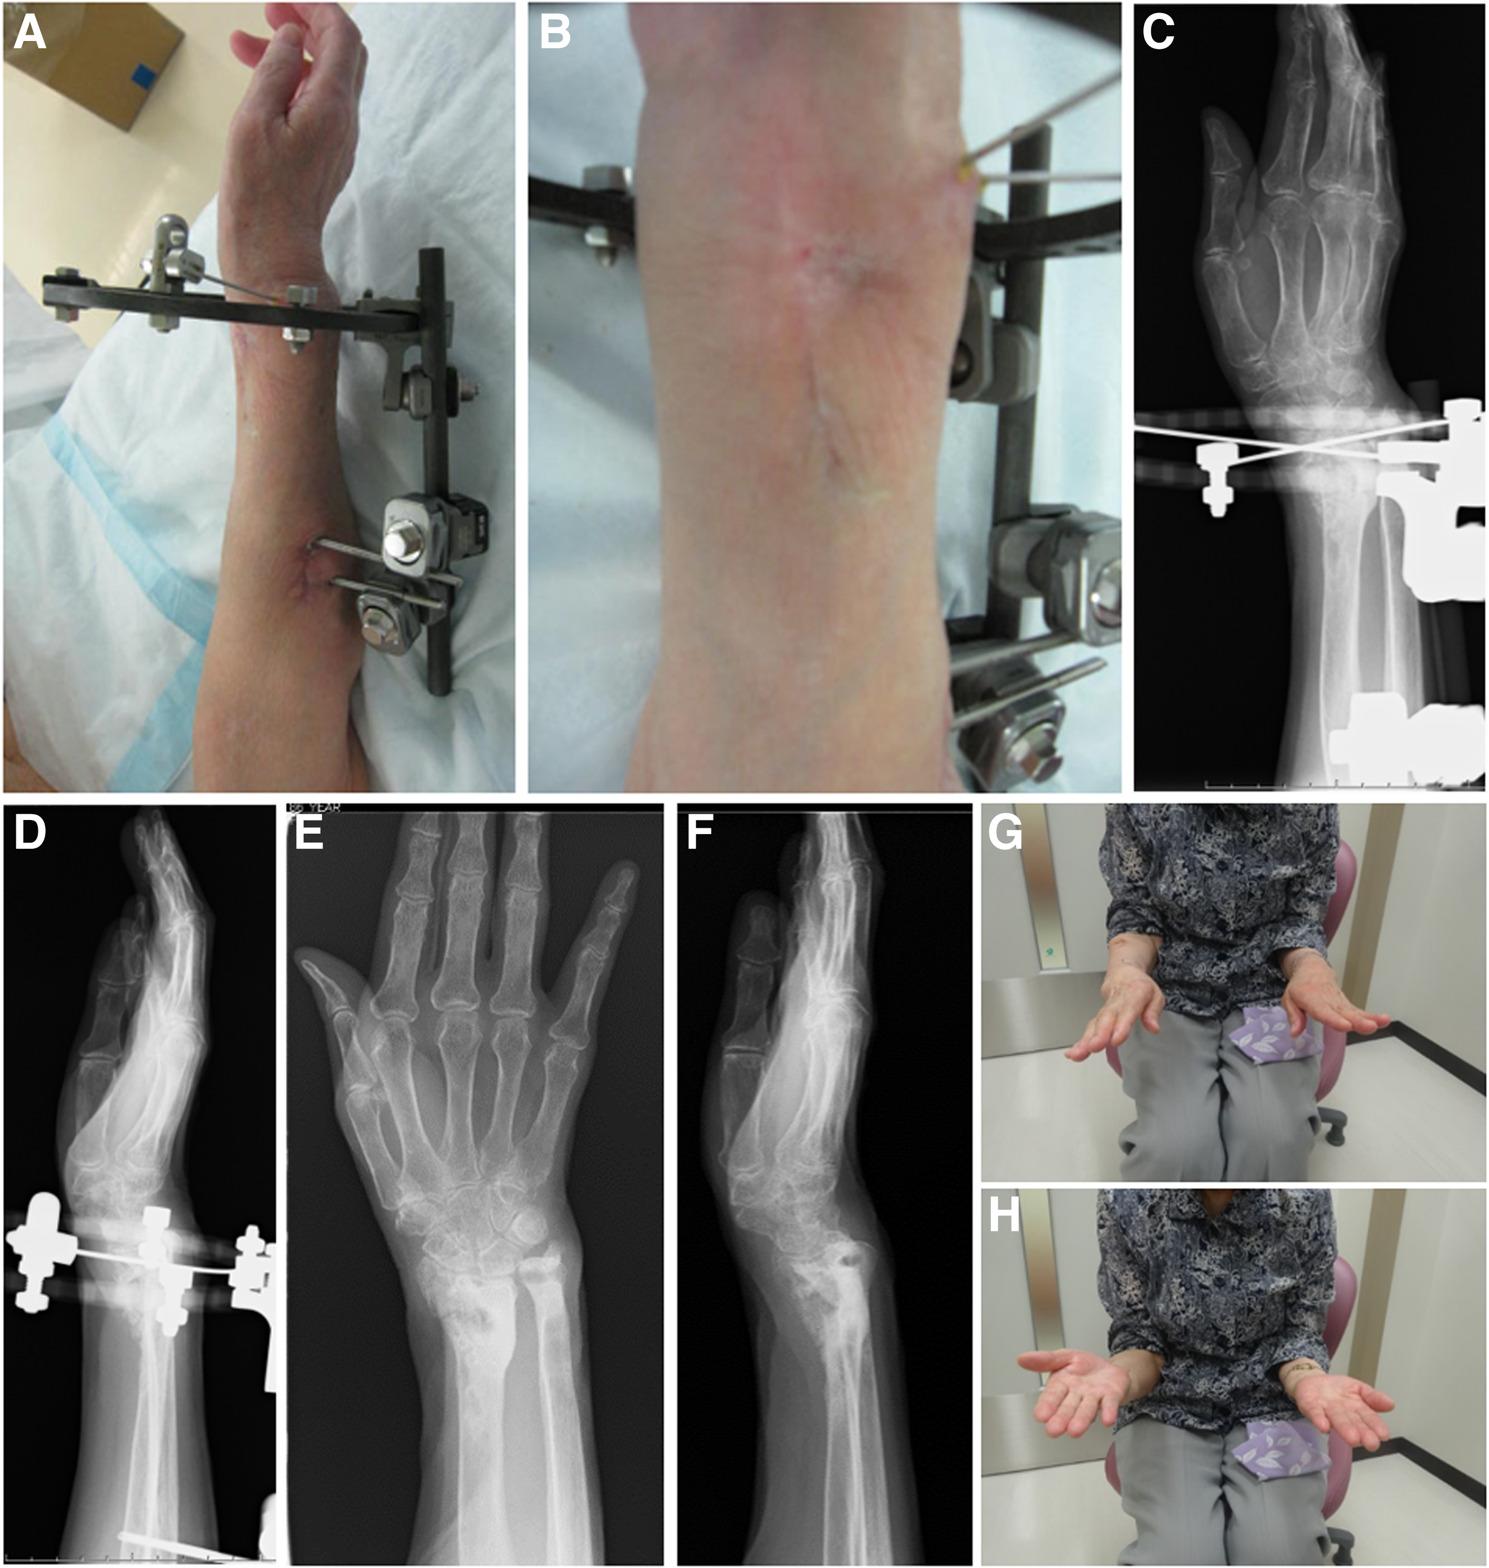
Clinical and radiographic findings after second-stage surgery and follow-up. A, B Healed sinus tract six weeks after first-stage surgery. C Anteroposterior radiograph after second-stage autologous cancellous bone grafting. D Lateral radiograph after second-stage surgery. E Anteroposterior radiograph demonstrating bony union prior to fixator removal. F Lateral radiograph confirming union. G Forearm pronation after fixator removal. H Forearm supination after fixator removal
